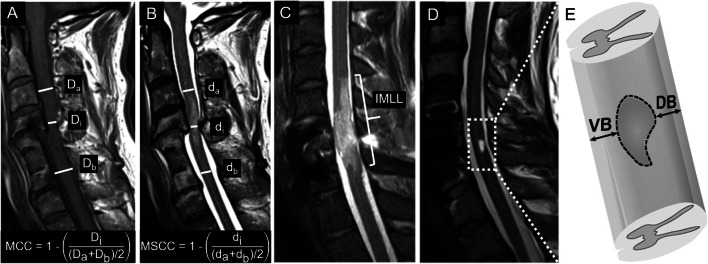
Lesion characteristics derived from standard MRI sequences. A Mid-sagittal T1-weighted MR image with the a-p diameters of the spinal canal at injury site (D_i_) and at nearest normal levels above (D_a_) and below (D_b_) the lesion, including the equation for MCC. B Mid-sagittal T2-weighted MR image with the a-p diameters of spinal cord at maximum injury site (d_i_) and at nearest normal levels above (d_a_) and below (d_b_) the lesion, including the equation for MSCC. C IMLL measured on a mid-sagittal T2-weighted MR image as the rostrocaudal length of the T2w hyperintense lesion. D Mid-sagittal T2-weighted MR image with a T2w hyperintense lesion for the determination of ventral and dorsal tissue bridges as indicated. Modified from Freund et al. [29]
